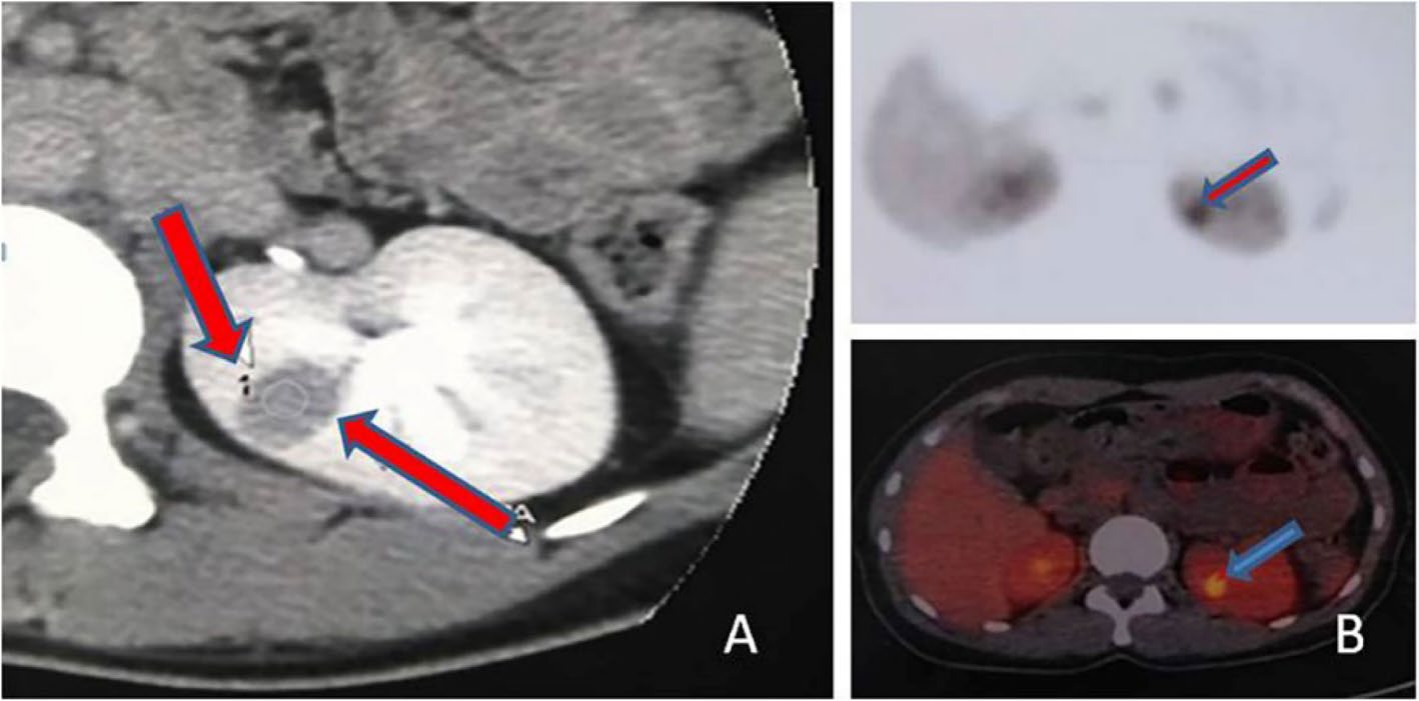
Abdominal contrast-enhanced CT and 18F-FDG PET-CT. A solid, circumscribed, low-density, cortical lesion (15 mm x13 mm) in the posterior lip of the left kidney, which appears to be moderately enhanced. There was no obvious enhancement in the arterial phase, but it was progressive in the venous phase and delayed phase. B mild FDG uptake in the mass
